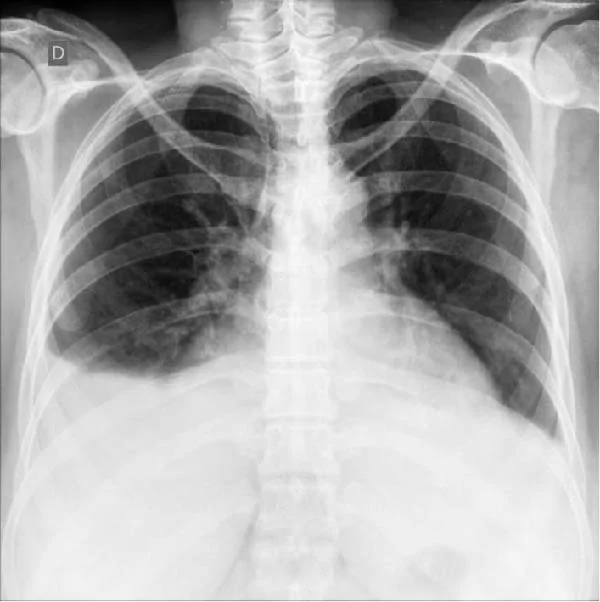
Posteroanterior chest radiograph with evidence of bilateral pleural effusion, 1 day after the first curettage.
